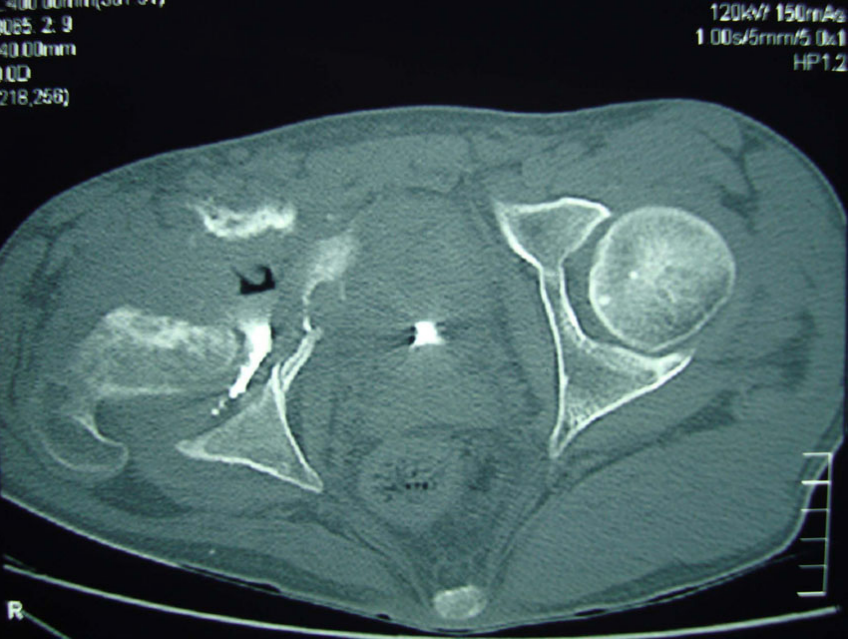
Axial computed tomography scan of the right acetabular cavity following retrograde urethrography showing destruction of the acetabular cavity and femoral head. Air-fluid level and contrast media accumulated around the femoral head.
